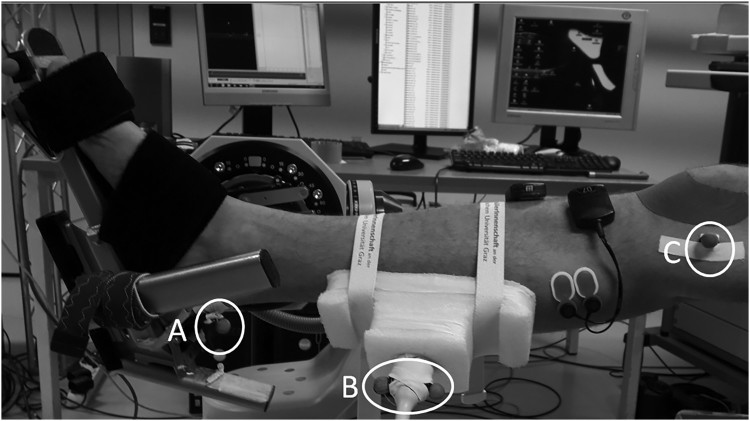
Experimental setup for the calculation of muscle and tendon lengths, with reflective markers on the calcaneus (A), two on the ultrasound probe (B), and on the medial epicondyle of the femur (C).
